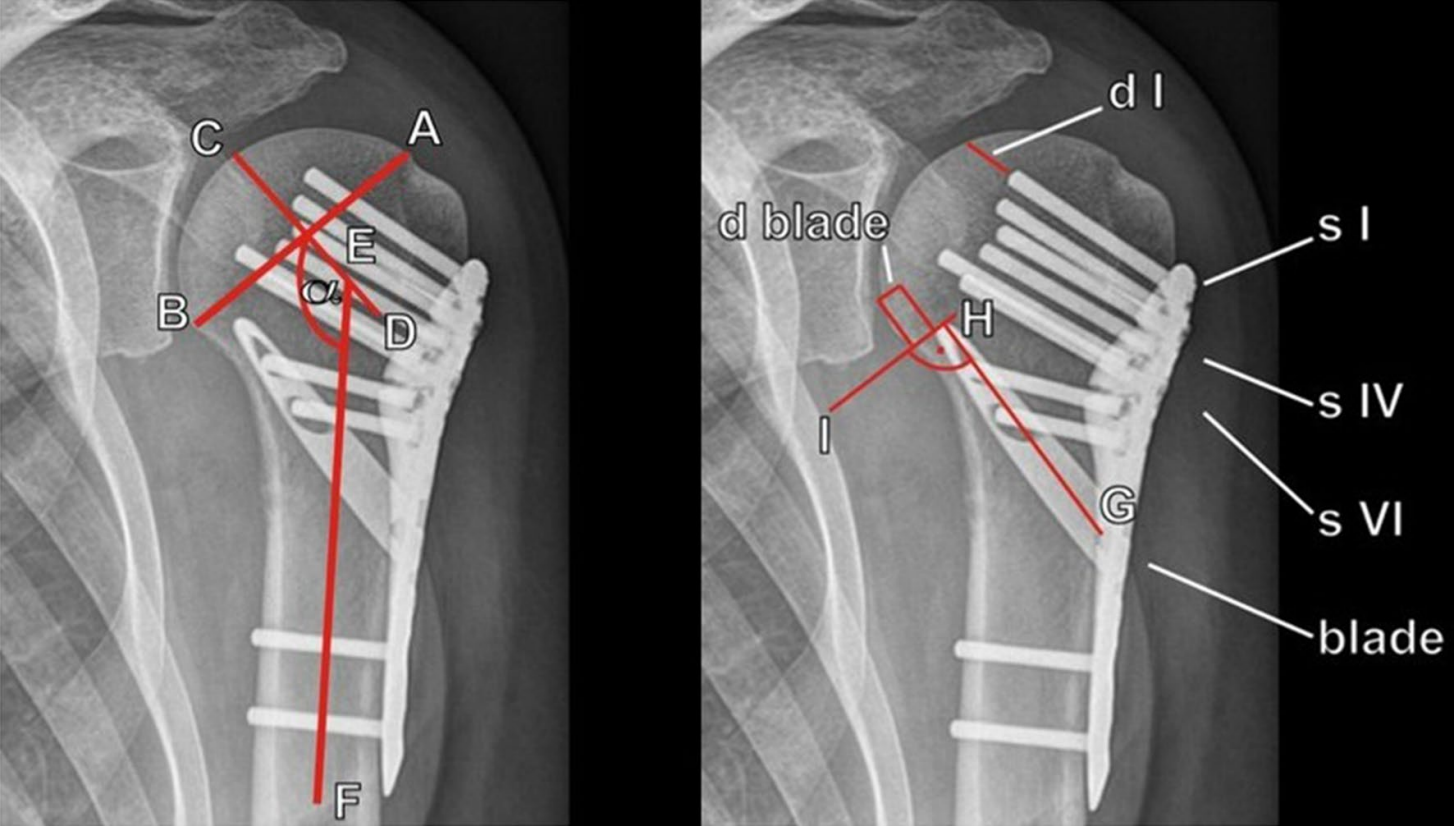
Radiographic evaluation. A line from the superior to the inferior border of the articular surface (*A*–*B*
